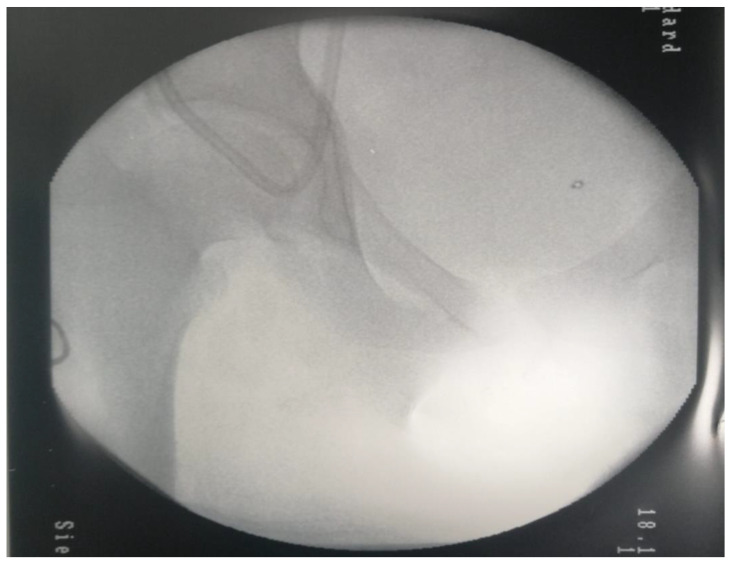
C arm X-ray—Unusual placement of CVC in the epigastric vein for chronic hemodialysis.
